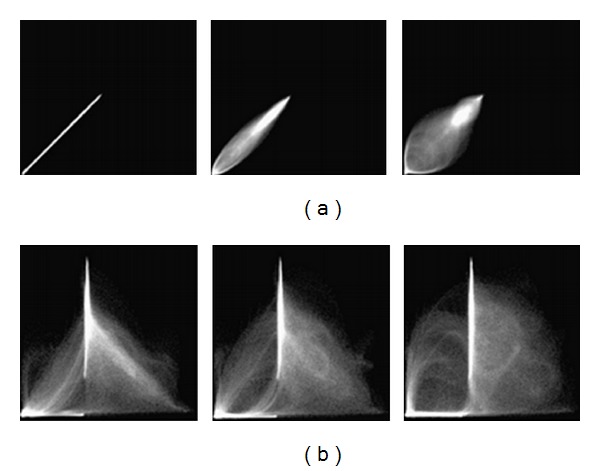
Example  2D histograms for (a) the same MR images of the head, (b) MR and CT images of the head. The left columns show two images when aligned, the middle columns show two image when translated by 2 mm, and the right columns show two images when translated by 5 mm. As can be seen, the joint histogram disperses with increasing mis-registration (obtained from Hill et al. (1994)).
